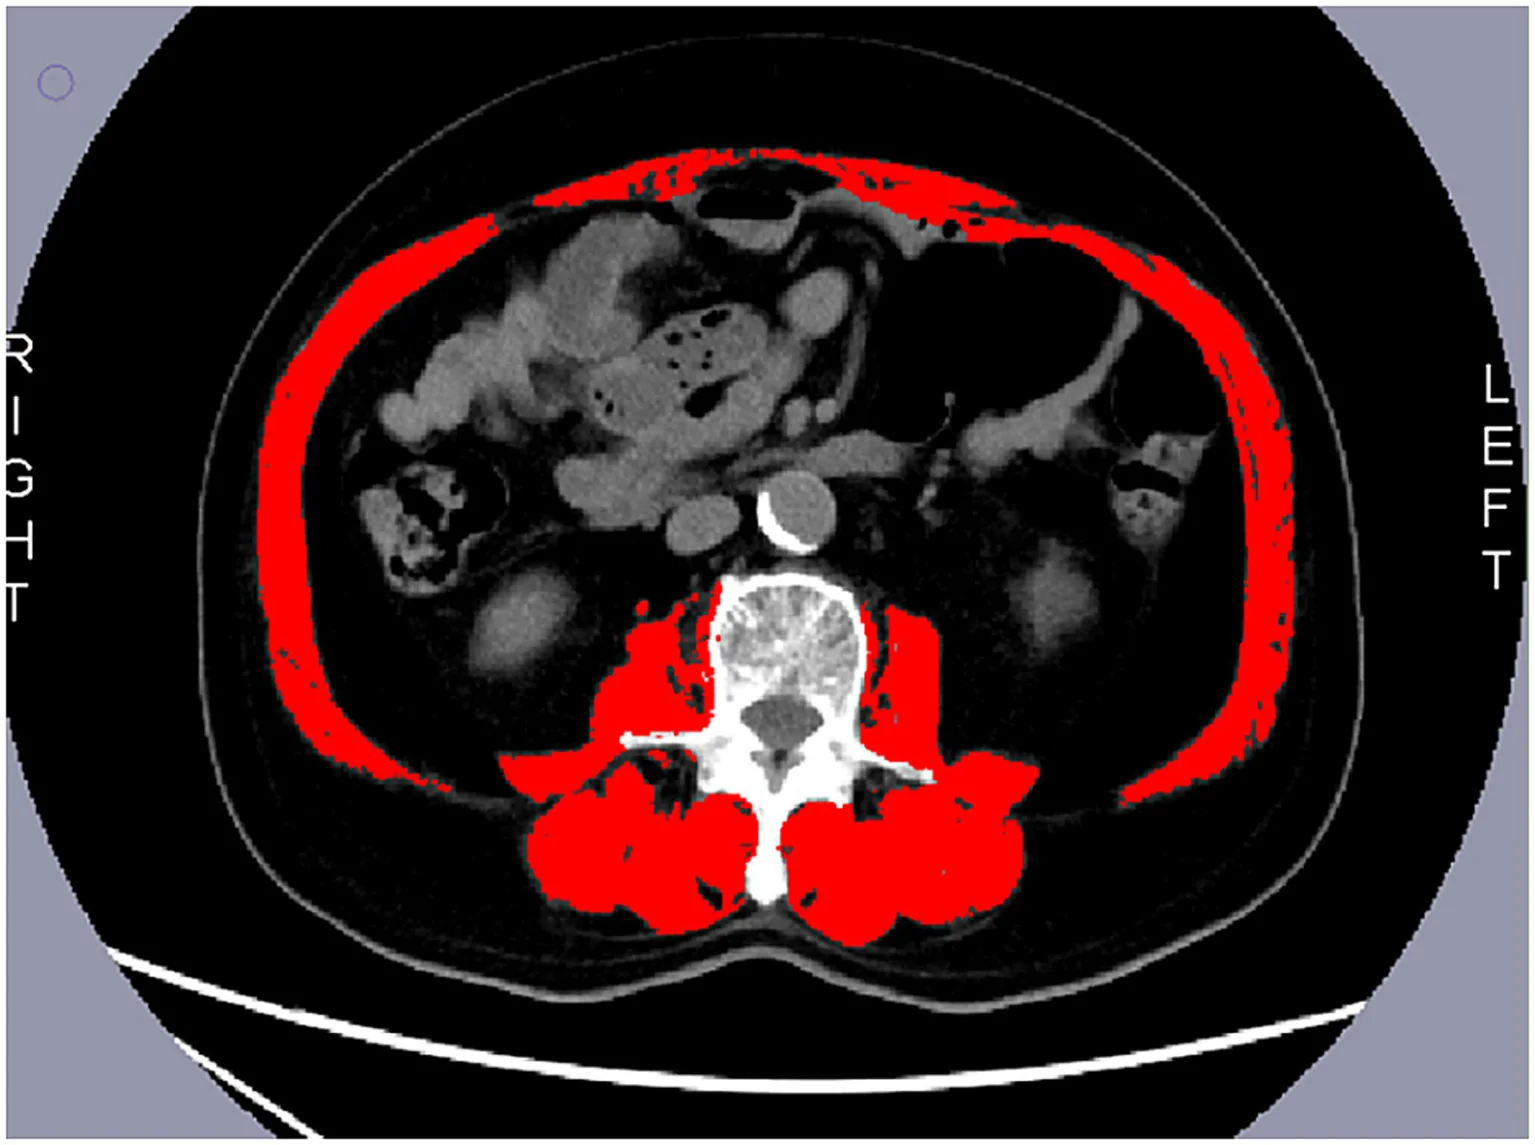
Representative axial computed tomography image at the level of the third lumbar vertebra for skeletal muscle analysis. The skeletal muscle area was 104.2 cm^2^, and the skeletal muscle index was 40.7 cm^2^/m^2^.
